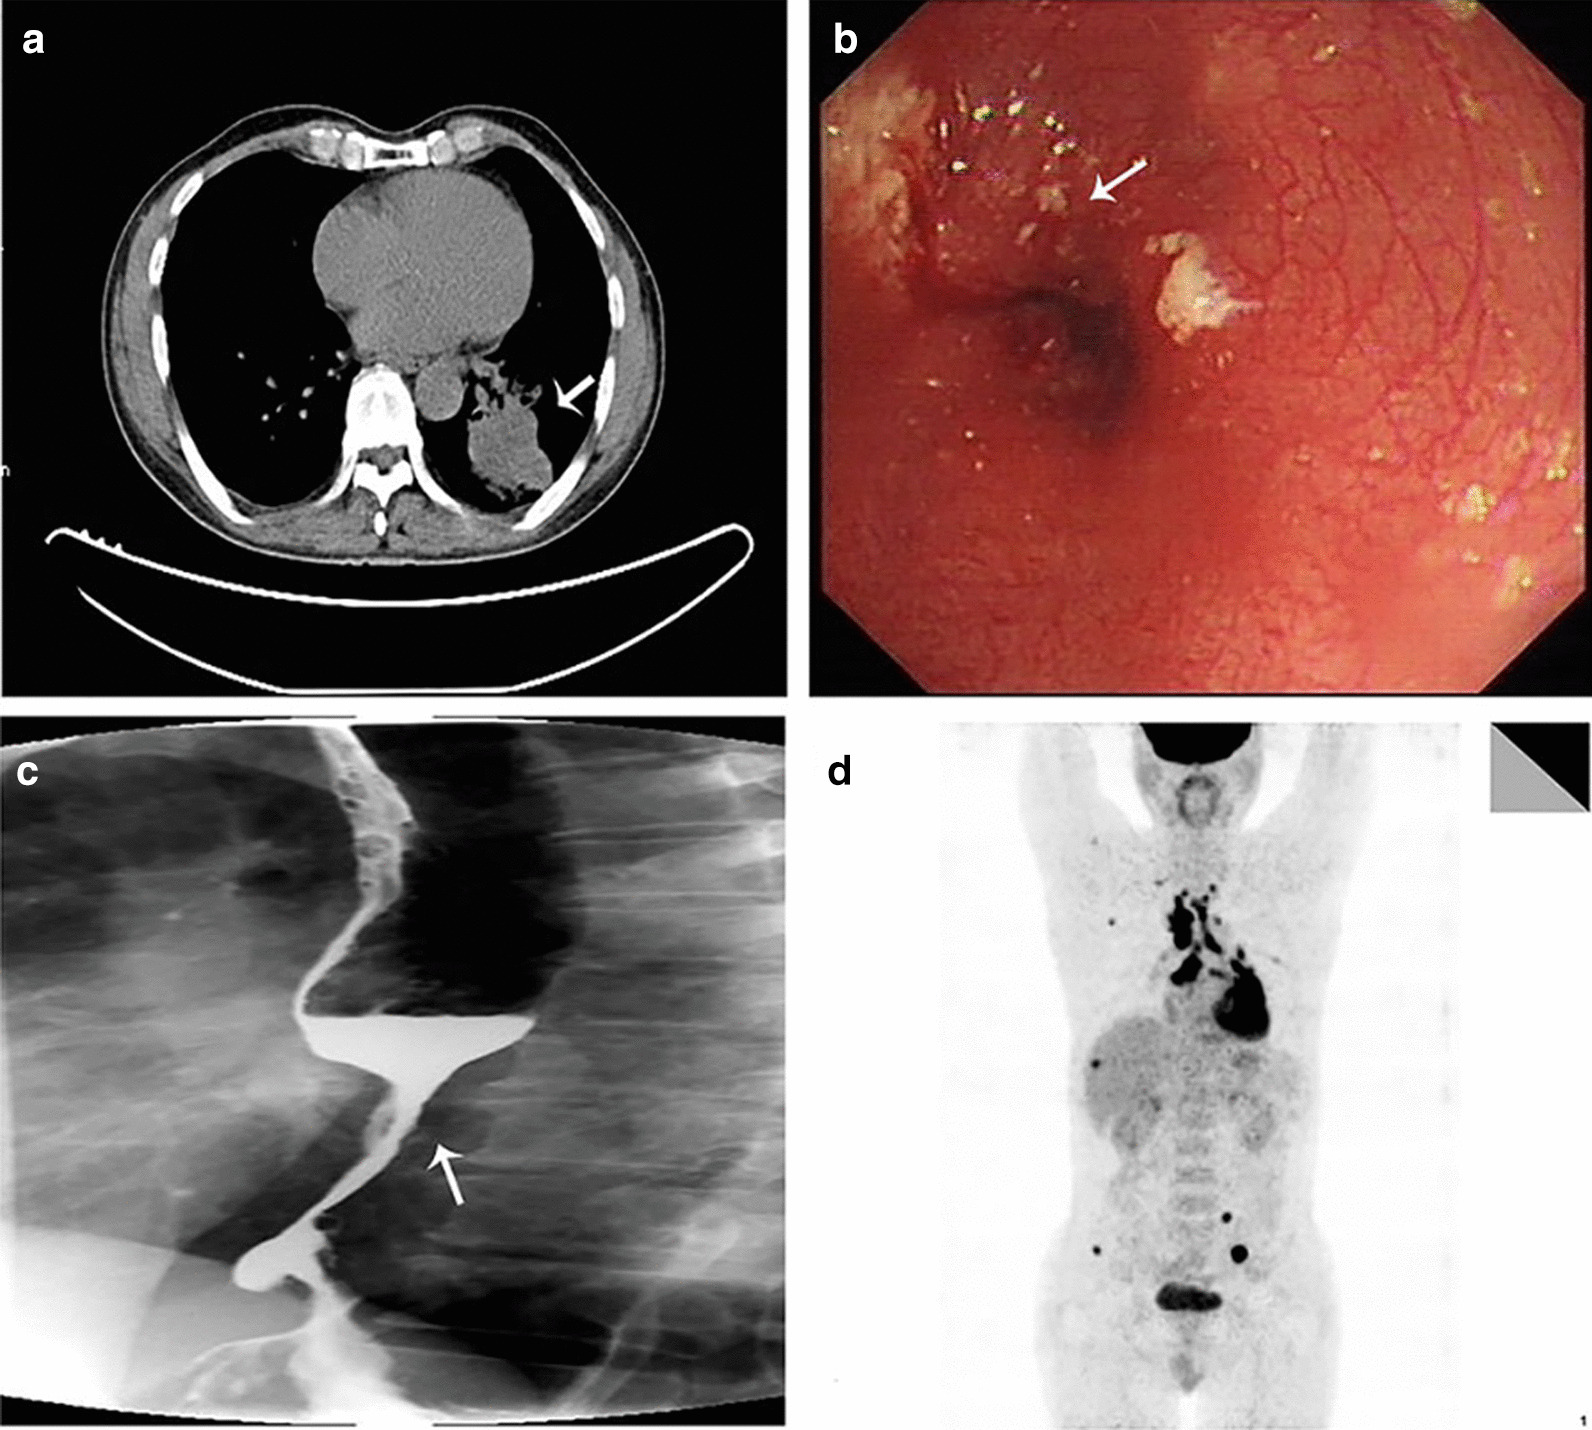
Imaging study of a patient with esophageal metastases from lung cancer. a Computed tomography (CT) scan of the chest showed a 48 × 42 mm defined tumor at the ascending left lung (arrow), with a scallop-shaped contour and focal enhancement. b Gastroscopy images showed a 17.7 mm wall thickening at the distal esophagus 38 cm from the upper incisors (arrow), with the mucous epithelium being mildly hemorrhagic but without a distinct break. c Barium esophagography. Esophagography showed irregular thickening of the wall and narrowing of the lumen of the lower thoracic esophagus (arrow). d Positron emission tomography (PET)-CT. PET-CT showed multiple metastases (lower left lung, bilateral supraclavicular fossa, hilus pulmonis, mediastinum lymph node, bone).
